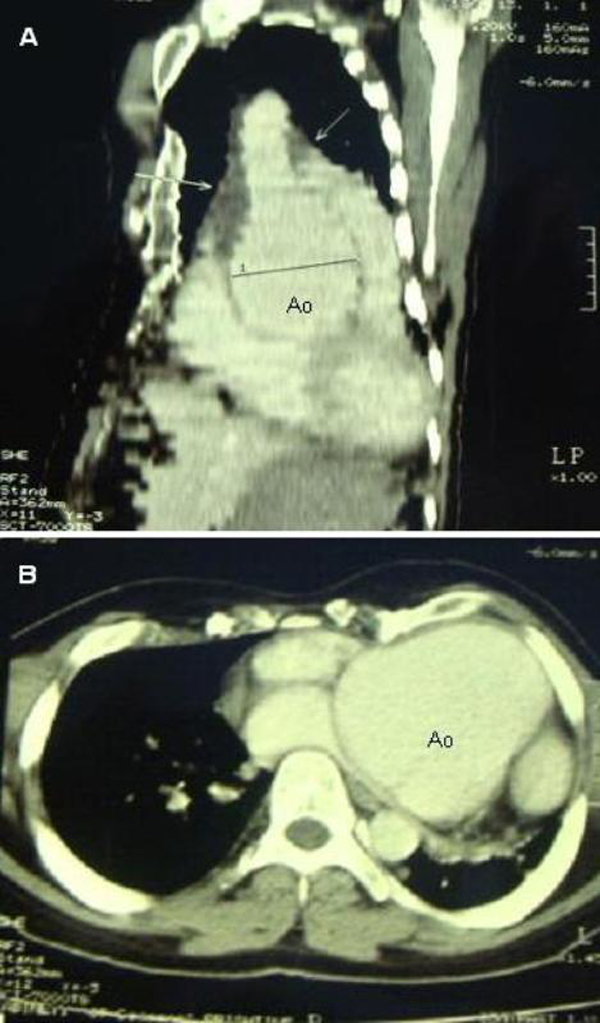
Chest CT. CT image in sagittal orientation showing a very large aortic root and intramural hematoma, which is depicted by arrows (A). Transverse CT image demonstrates a large aortic bulb and heart structures encompassing almost entire left hemi-thorax (B).
